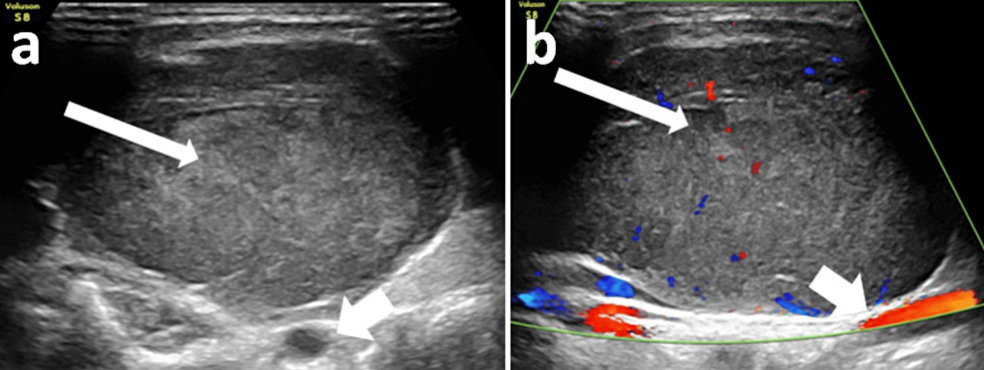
Ultrasound images of the neck demonstrating a lesion anterior to the right internal carotid artery. (a) USG neck (longitudinal orientation) showing relatively heterogenous lesion (long white arrow) anterior to the right internal carotid artery (short white arrow). (b) USG neck colour Doppler shows mild internal vascularity within the lesion (long white arrow) and colour uptake within the internal carotid artery (short white arrow) (Probe marker: Voluson S8).
